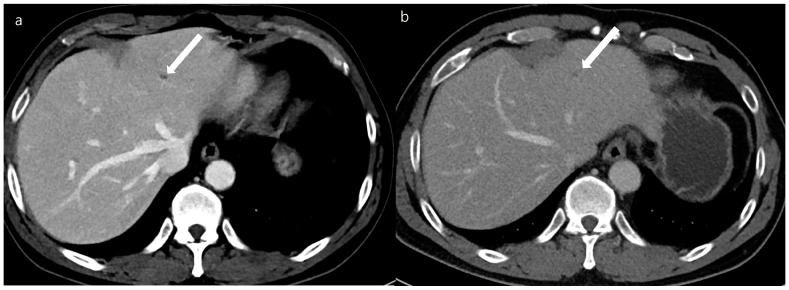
A 54-year-old man with a 0.4 cm sized small focal low density at segment 2 of the liver (arrows). A double low-dose CT using a deep-learning-based contrast-boosting model (a) shows better conspicuity (Reviewer 1, 4; Reviewer 2, 5) of the focal lesion (arrows) than standard-dose CT using hybrid iterative reconstruction (Reviewer 1, 2; Reviewer 2, 1) (b) at portal phase images (time interval: 11 months). Two reviewers evaluated the double low-dose CT using a deep-learning-based contrast-boosting model and standard-dose CT using hybrid iterative reconstruction. The results demonstrated no difference in overall image quality.
